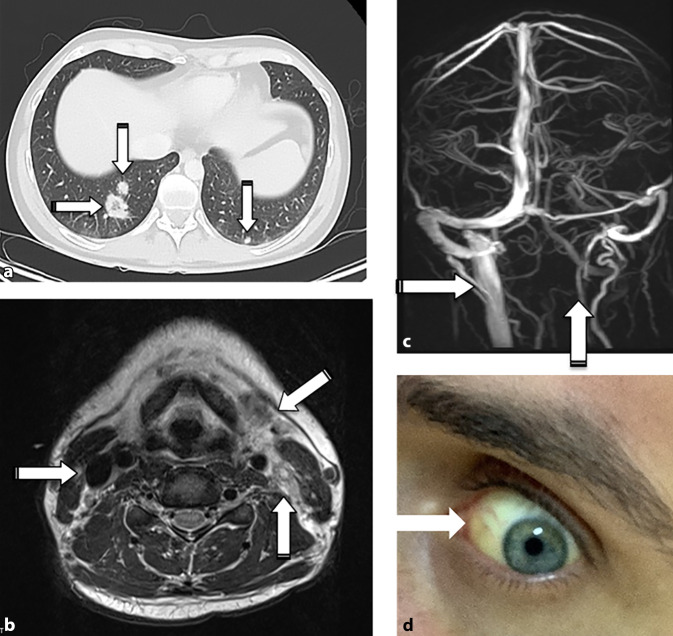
a CT der Lunge mit bilateralem Nachweis multipler, a.e. septischer Embolien (*Pfeile*). b Axiale, T2-gewichtete Turbo-Spin-Echo-Sequenz des Halses: Thrombose der V. jugularis sinistra wegen fehlendem Flow-Void (*vertikaler Pfeil*), regelrecht durchflossene V. jugularis dextra (*horizontaler Pfeil*). Lymphadenopathie (*schräger Pfeil*). c Koronare Maximumintensitätsprojektion der 3D-Phasenfllussmessung der zerebralen Sinus und Venen mit ausbleibendem Signal in der okkludierten V. jugularis sinistra (*vertikaler Pfeil*) und regelrechter Kontrastierung kontralateral (*horizontaler Pfeil*) sowie der Sinus. d Klinisches Bild bei Untersuchung auf Intensivstation mit Sklerenikterus (*Pfeil*)
